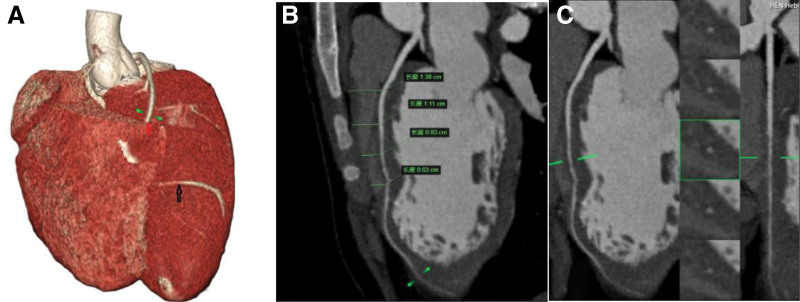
Coronary computed tomography angiography. (A) The red arrow represents the site where the myocardial bridge perforates the myocardium, and the black arrow represents the site where the myocardial bridge is close to the myocardium. (B) Represents the depth of the myocardial bridge at different locations. (C) The anterior descending coronary artery ran completely between the left and right ventricles.
